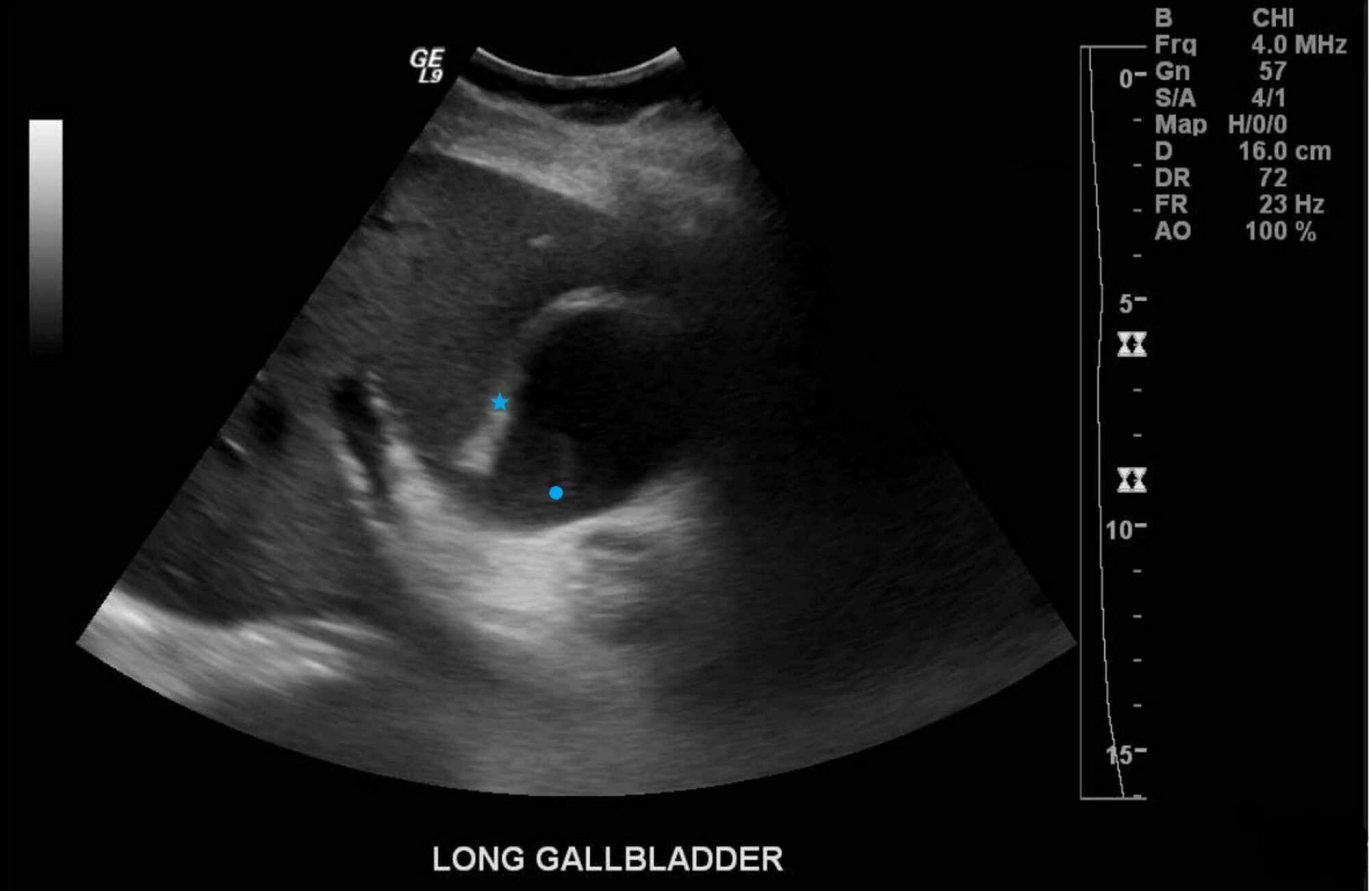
Ultrasound of the abdomen demonstrating gallbladder wall thickening (star) and layering sludge (circle), concerning for acute cholecystitis
